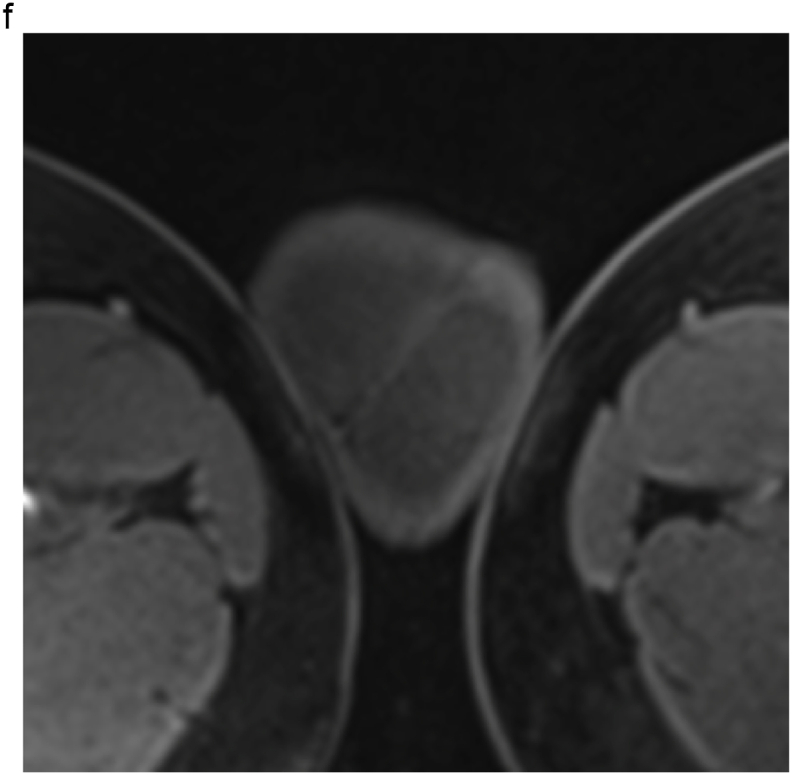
(a) T2-weighted MRI taken at 4 months after the initiation of conservative syphilis treatment showing that the mass had decreased in size. (b) At 4 months, the contrast enhancement had decreased markedly. (c) MRI taken at 10 months after the initiation of treatment. (d) At 10 months, the contrast enhancement remained decreased. (e) MRI taken at 16 months after the initiation of treatment. On T2-weighted images, the mass persisted. (f) At 16 months, no contrast enhancement was observed.
